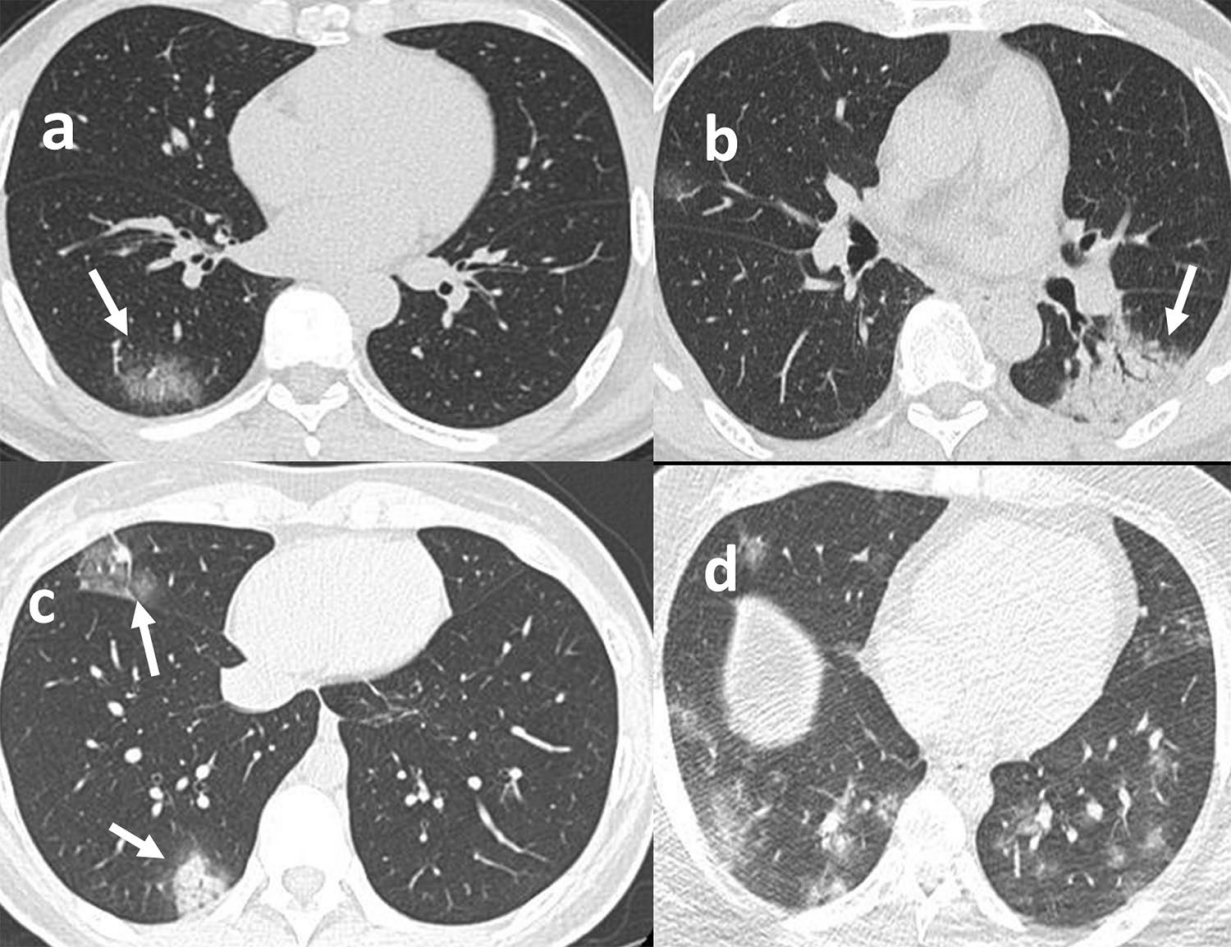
Chest CT findings of COVID-19 pneumonia. (a) GGO; (b) consolidation; (c) multifocal lesions; (d) bilateral lesions.
